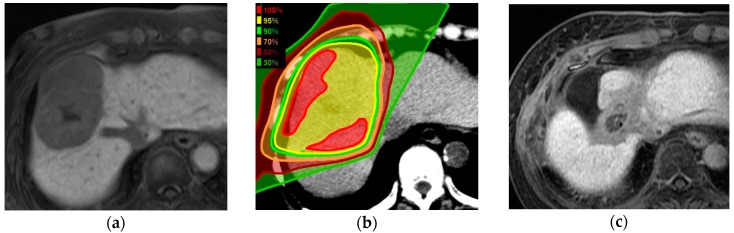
HCC (7.9 × 5.6 cm) treated by PBT (66 CGE/10 fractions) in a female in her eighties with hepatitis C liver cirrhosis, Child–Pugh class A. Tumor shows hypo-intensity in the hepatobiliary phase of gadolinium ethoxybenzyl diethylenetriamine pentaacetic acid-enhanced MRI (EOB-MRI) before treatment (a). The isodose lines displayed on planning CT (b). Three years on from PBT, the tumor was reduced in size on EOB-MRI. The normal liver in the irradiated area was atrophied, but severe liver damage was not observed (c).
